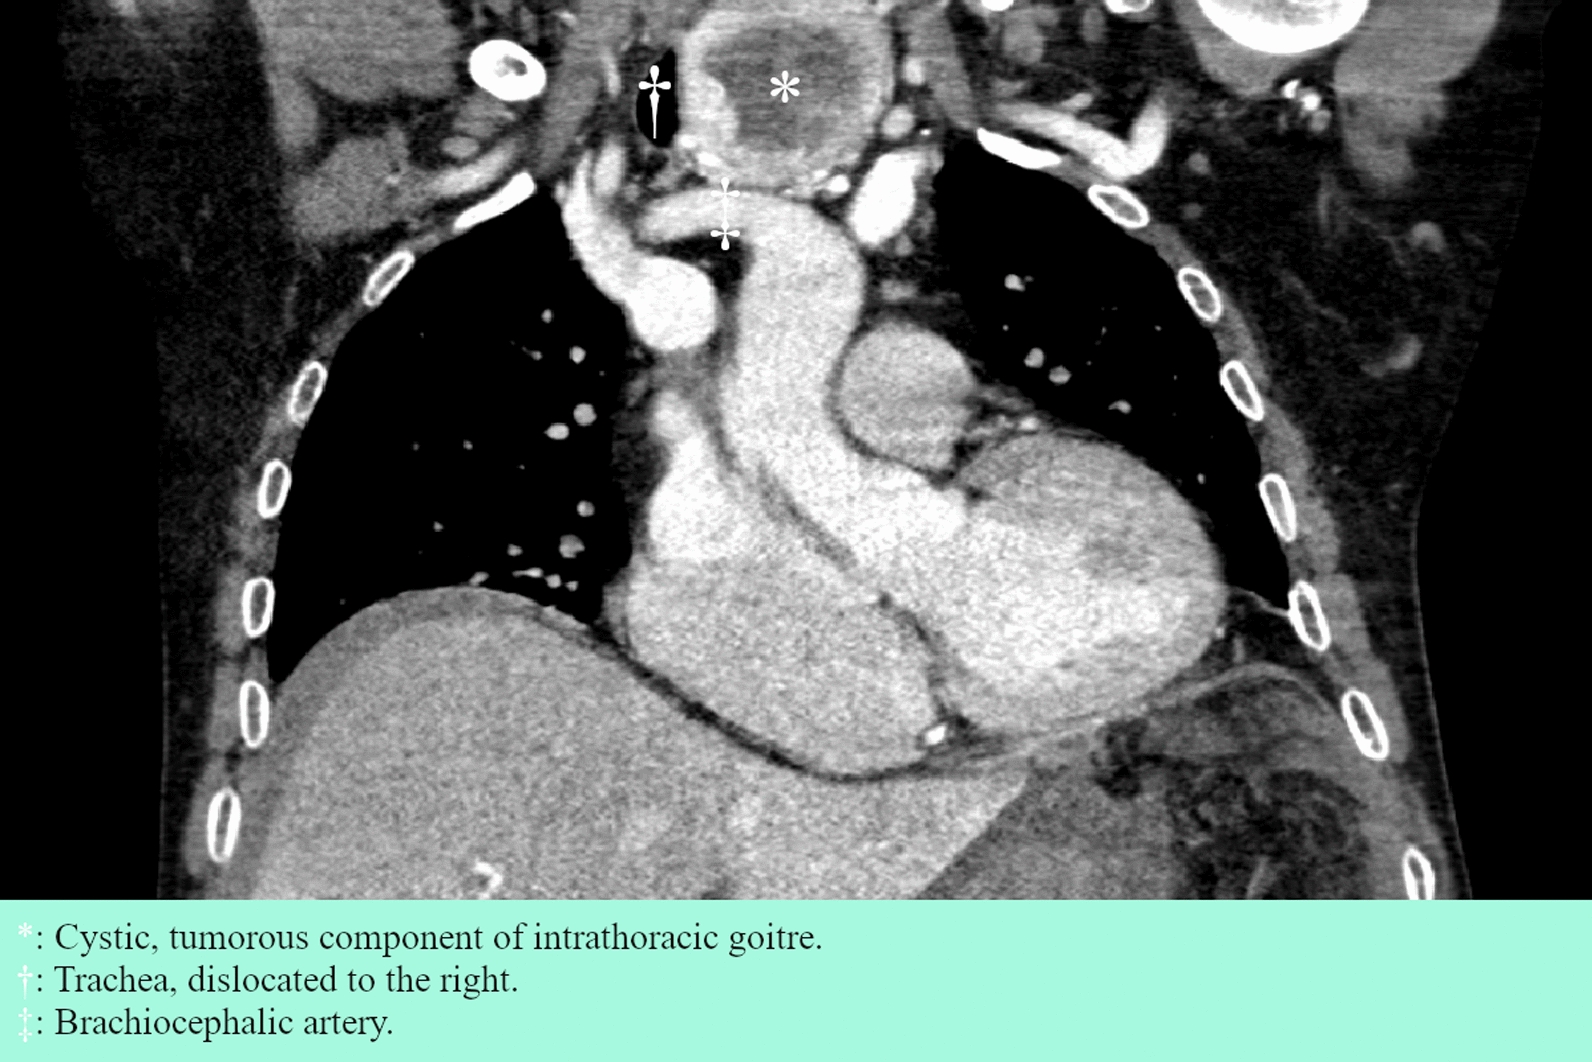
Coronal section of thoracoabdominal CT scan on the fourth day after admission
